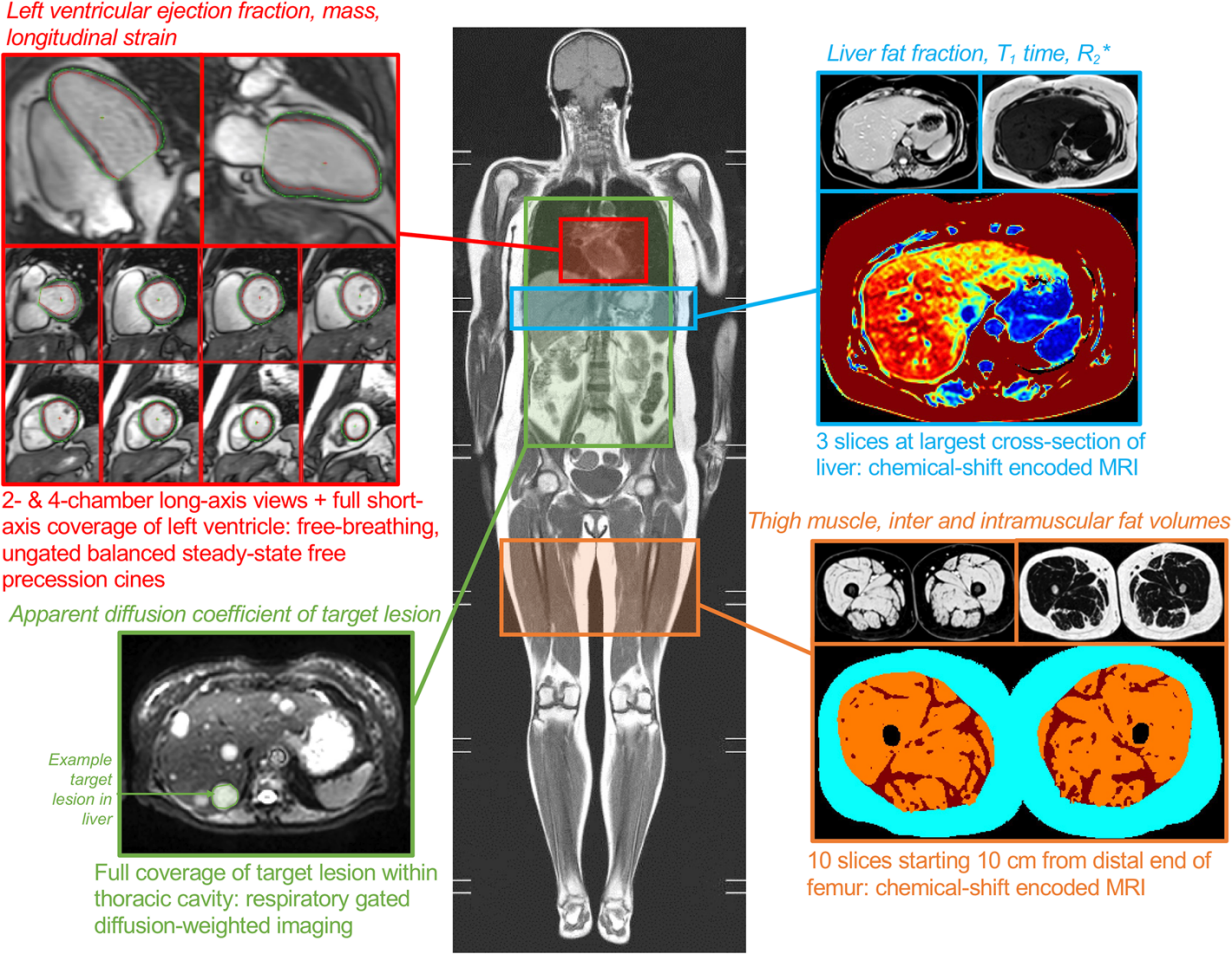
Magnetic resonance imaging protocol
